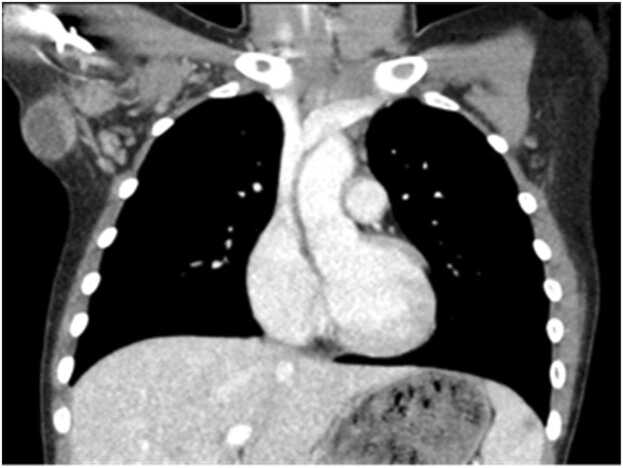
Case 7. CT thorax/abdomen with peripherally enhancing bilobed fluid collection in the right axilla.
